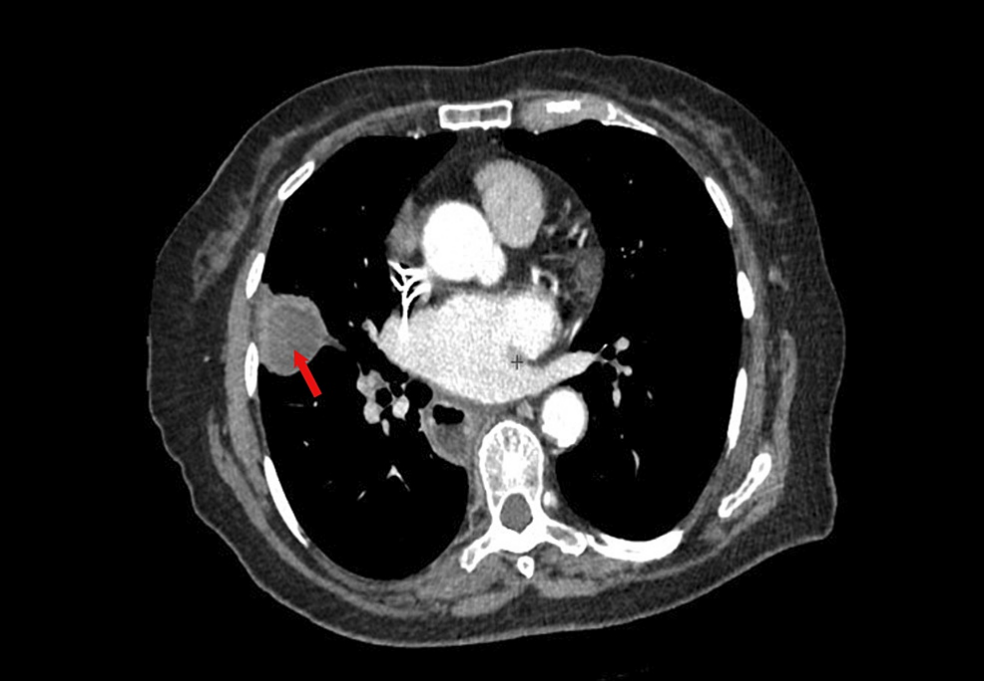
Thoracic CT scan (mediastinal window) showing a 3 x 3 x 2cm spiculated nodule (red arrow) of the right upper lung lobe.
